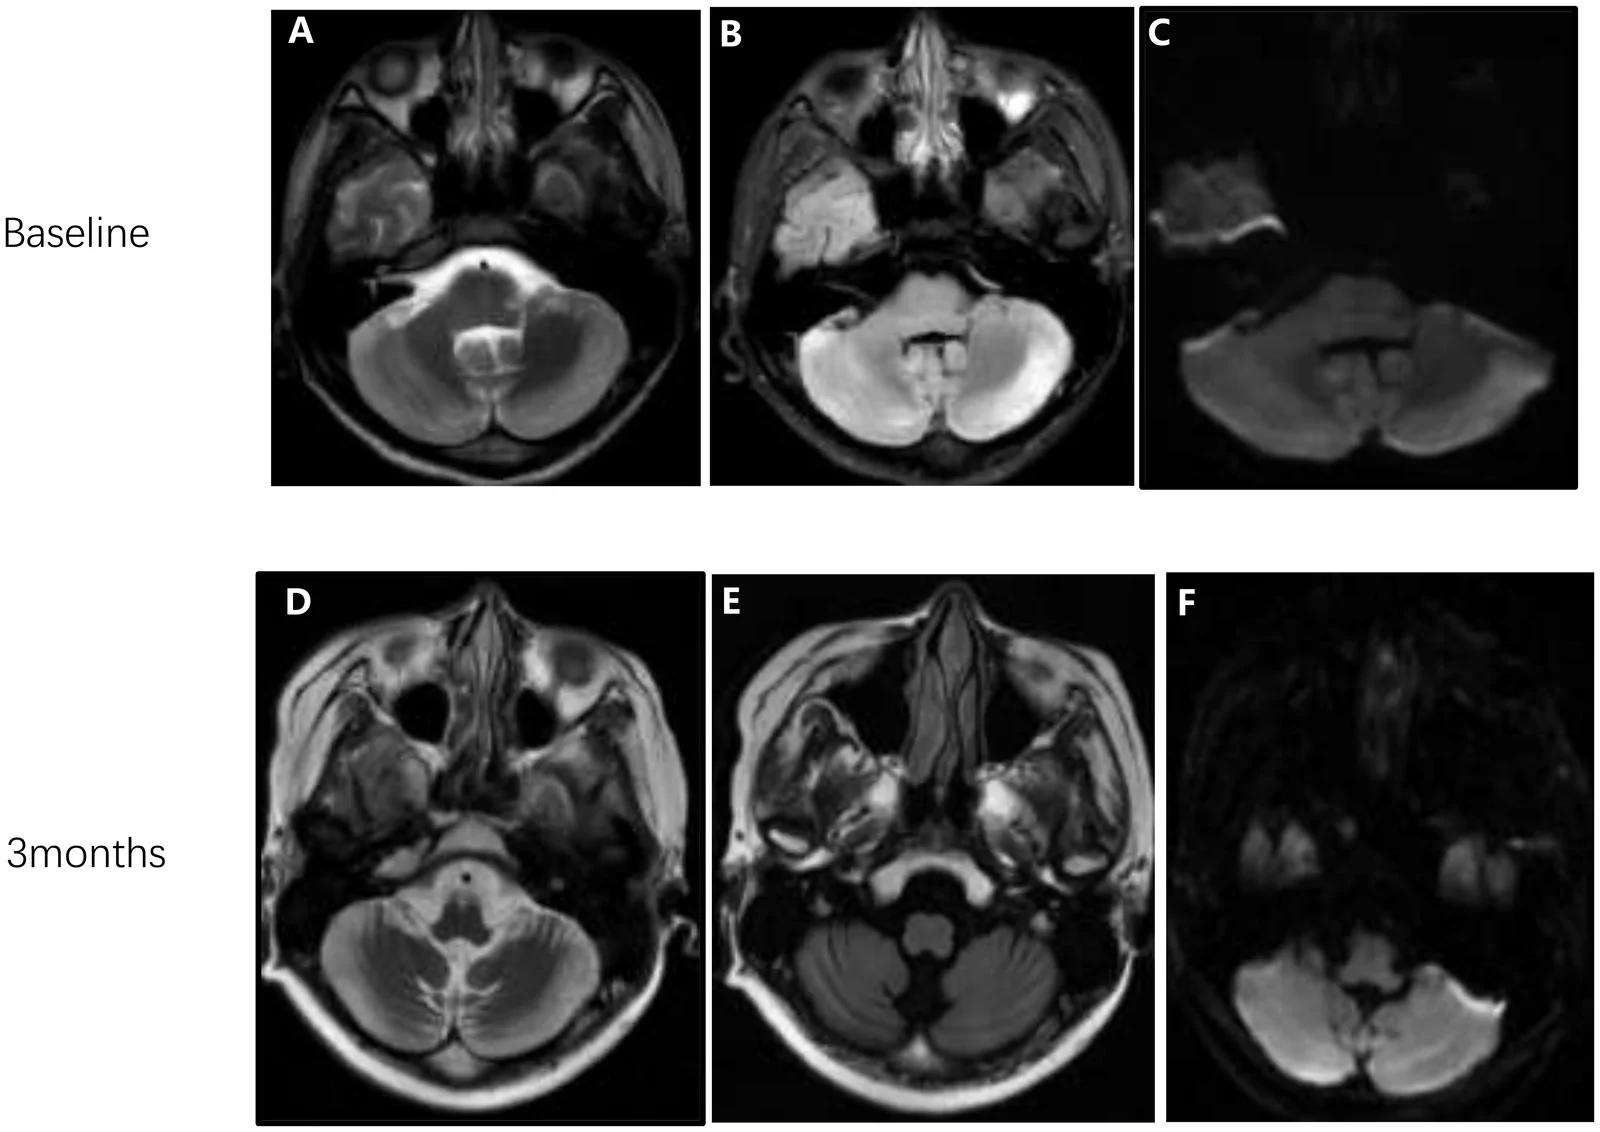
Magnetic resonance imaging (MRI) of the brain showed no abnormal signs. (A–C) T2WI, T2-FLAIR, and DWI axial views of the temporal lobe and cerebellum. T2WI, T2-weighted imaging; FLAIR, T2-weighted fluid-attenuated inversion recovery; DWI, diffusion-weighted imaging. (D–F) Showed mild bilateral cerebellar hemisphere atrophy.
